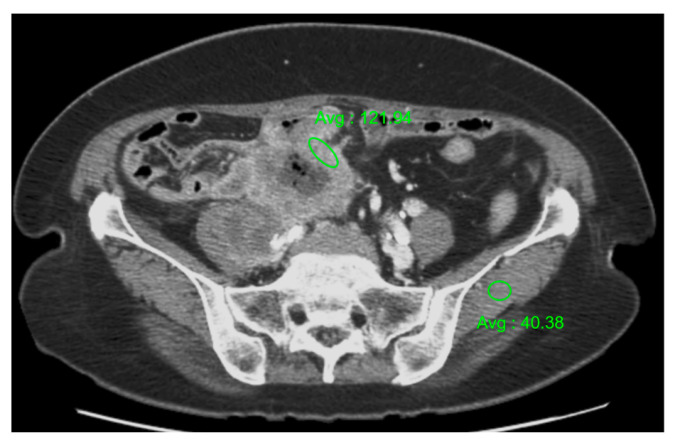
A CT image of the method used to measure the ratio of maximum contrast enhancement between the tumor and the back muscle in the same phase of a contrast-enhanced CT scan. A ratio of 3.01, as shown, is categorized as a good enhancement level (ratio > 1.5).
